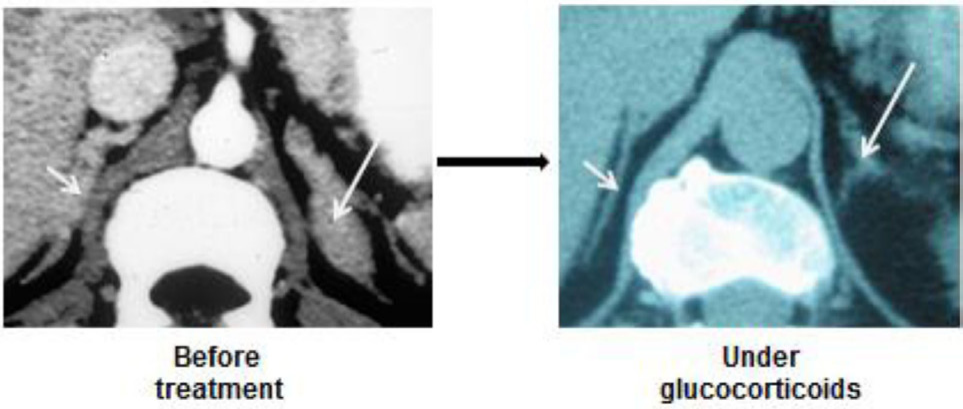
CT-scan images of enlarged and nodular adrenal glands in a patient with 21 hydroxylase deficiency. Regression under ACTH suppression by large doses of glucocorticoids.
